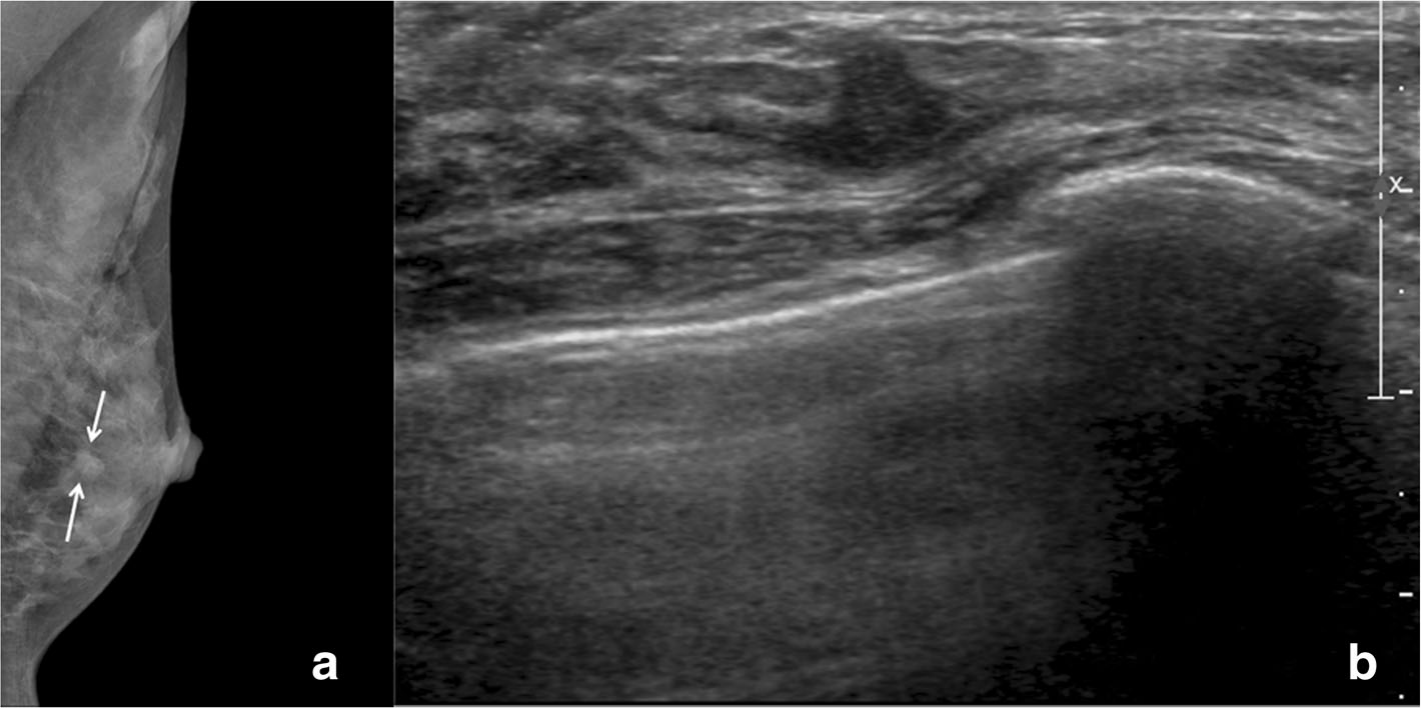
A radial scar on ultrasound guided core needle biopsy and upgraded to radial scar with atypical ductal hyperplasia after surgical excision. a On mammography, there is an irregular, indistinct, hyperdense mass (arrow). b On ultrasound, there is an irregular, non-parallel, angular, hypoechoic mass
